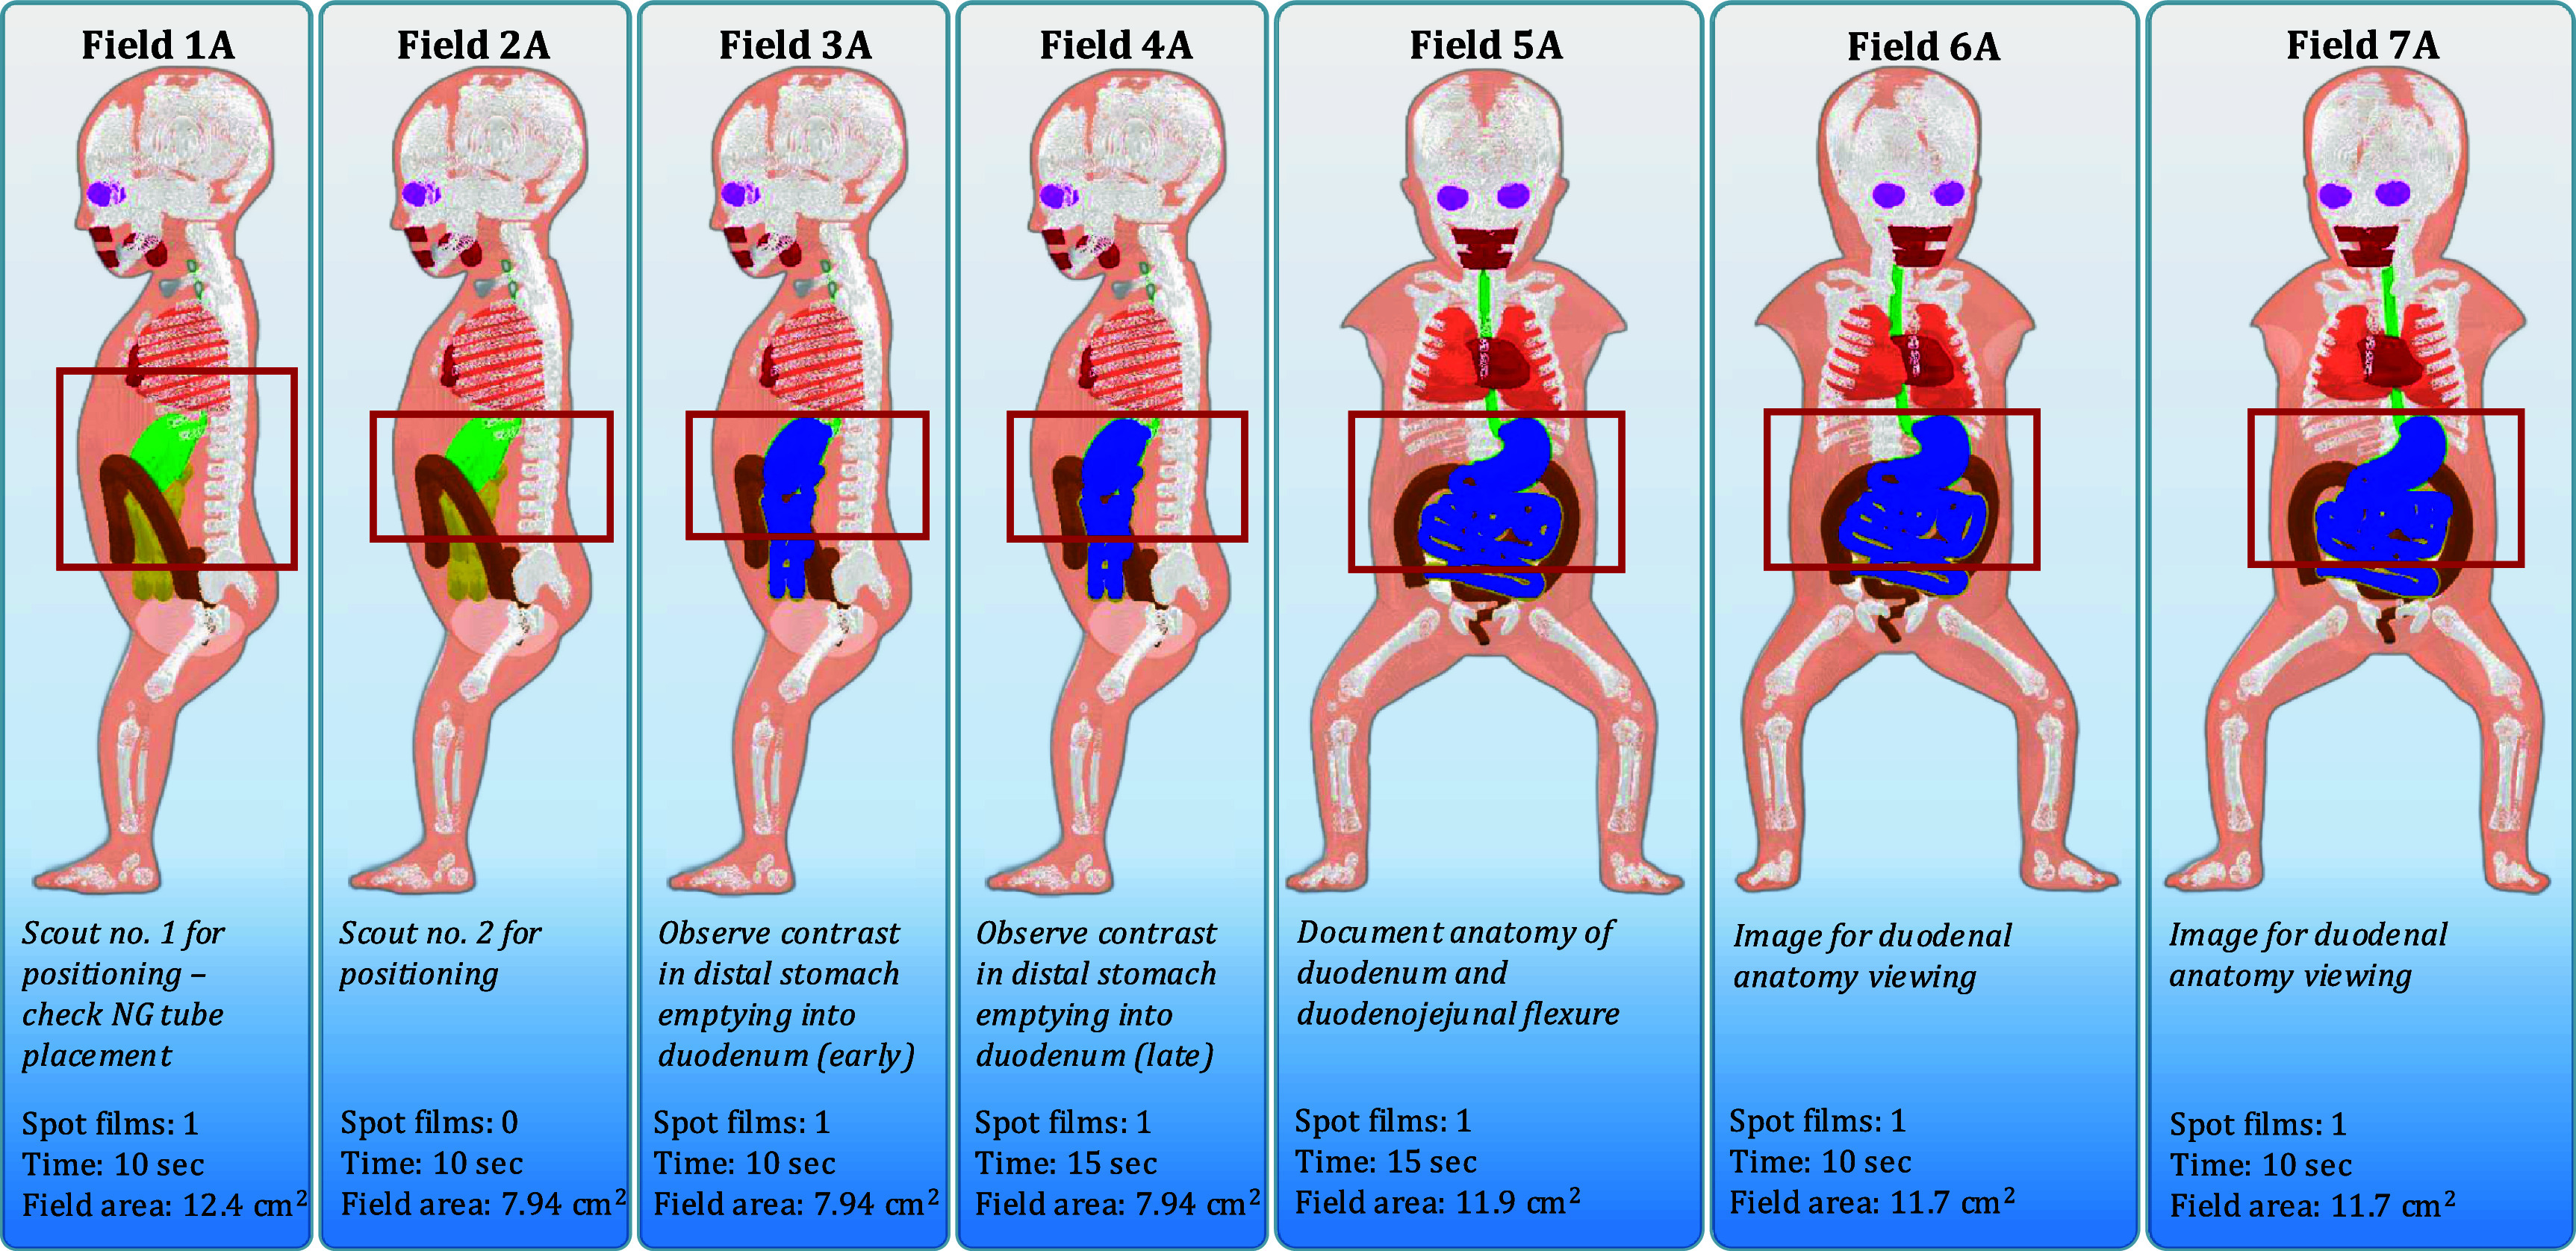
Examination outline with demarcated fields for a newborn female undergoing a UGI series resulting in an abnormal diagnosis. Field area calculated at 20 cm from the x-ray source.
